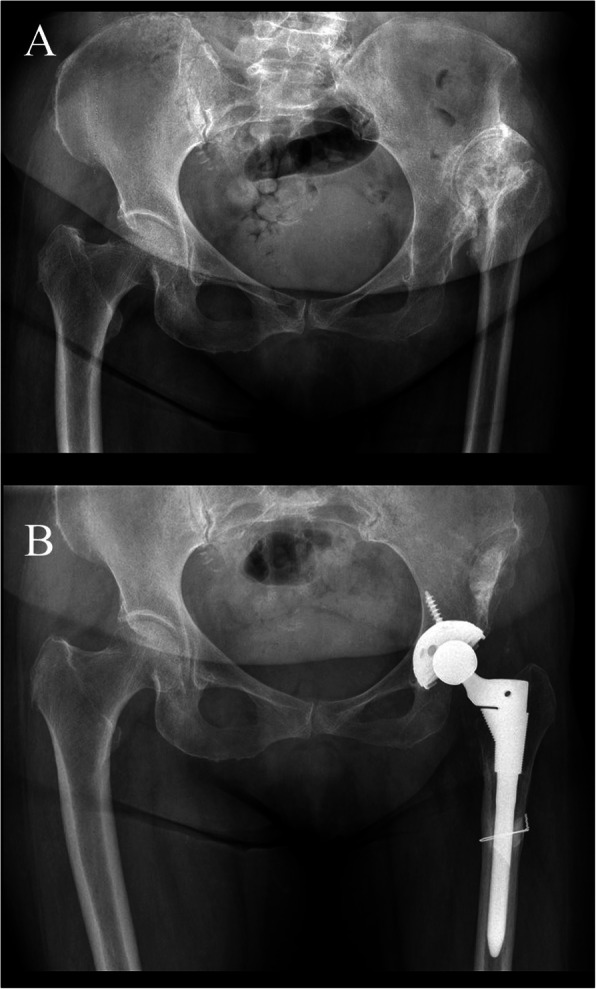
A 71-year-old woman with left high hip dislocation. Preoperative (a) and postoperative (b) radiographs at 5-year follow up
